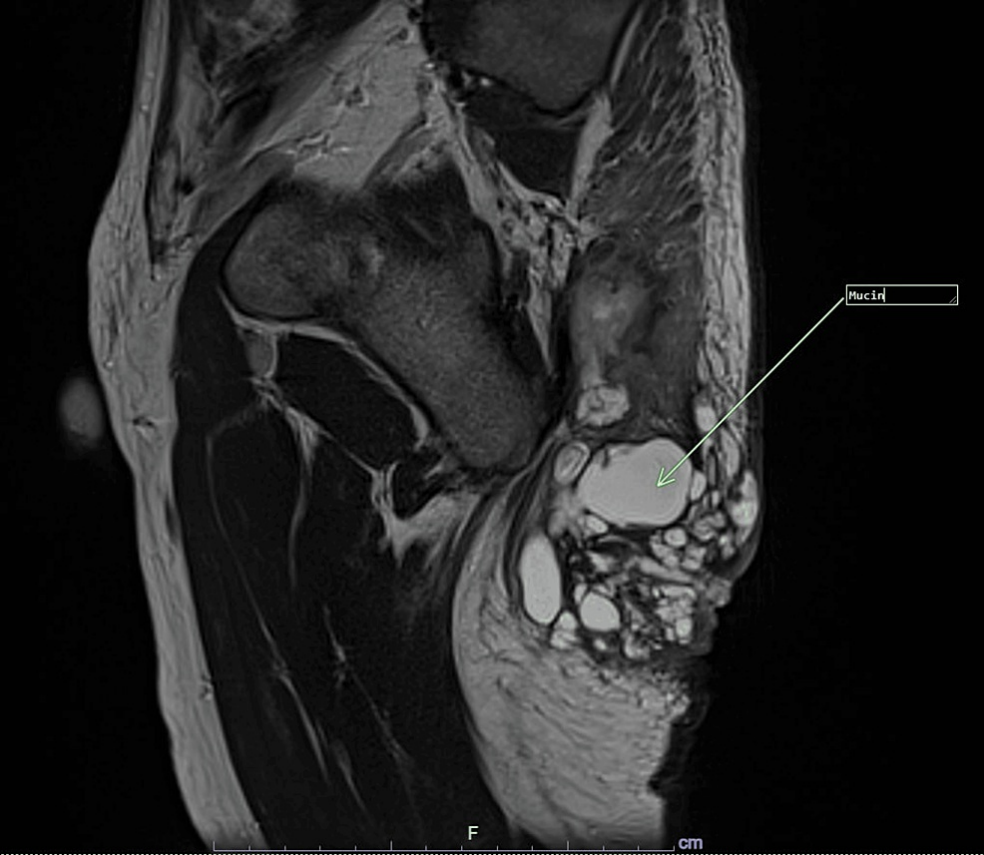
MRI (sagittal section): A complex, multiloculated collection containing mucin communicating with the right buttock was demonstrated.
